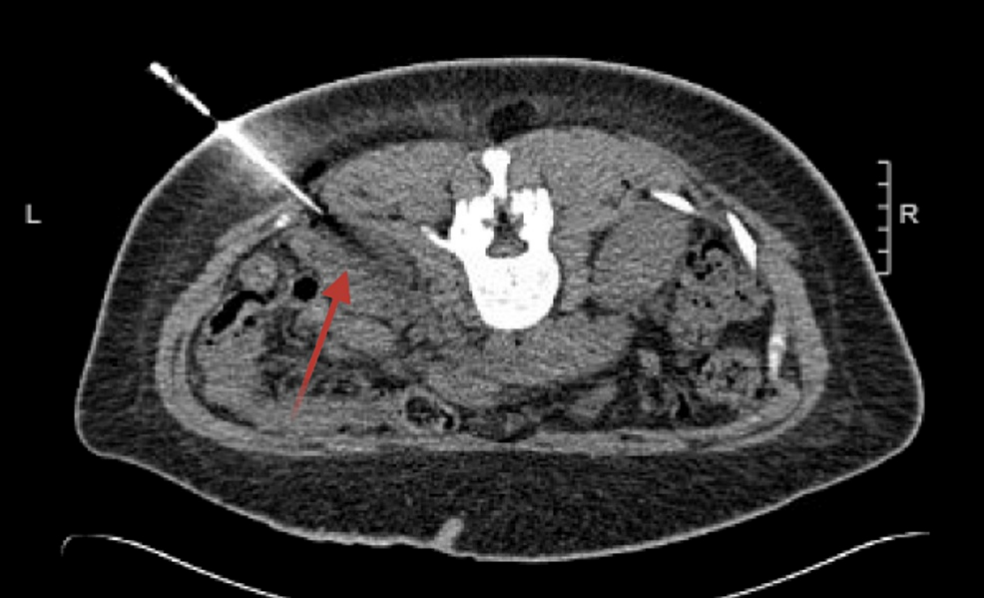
Technically successful CT-guided native left renal biopsy (arrows pointing to the site of the biopsy).
